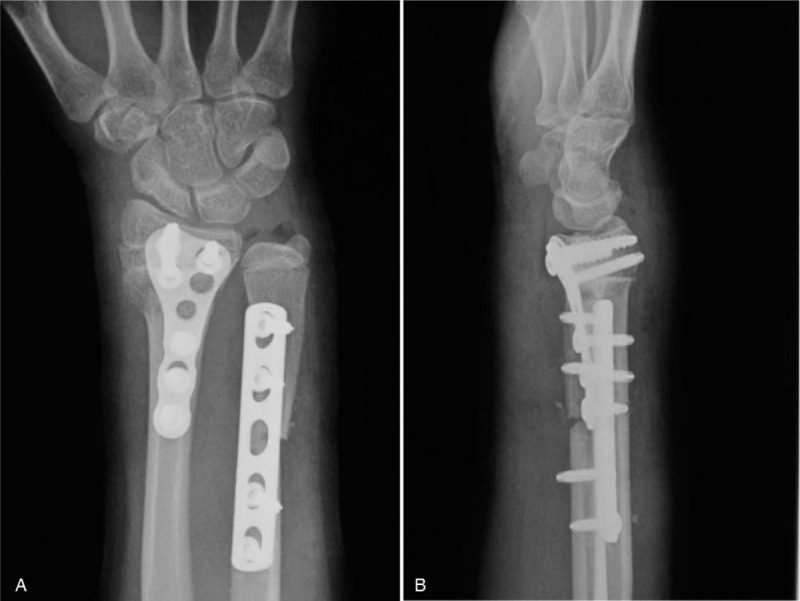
Postoperative radiographs of the right wrist. (A) Anteroposterior view. (B) Lateral view. The dorsal angulation of the distal radius was corrected and fixed with a volar locking plate. Ulnar shortening osteotomy was performed, followed by fixation of the locking plate.
